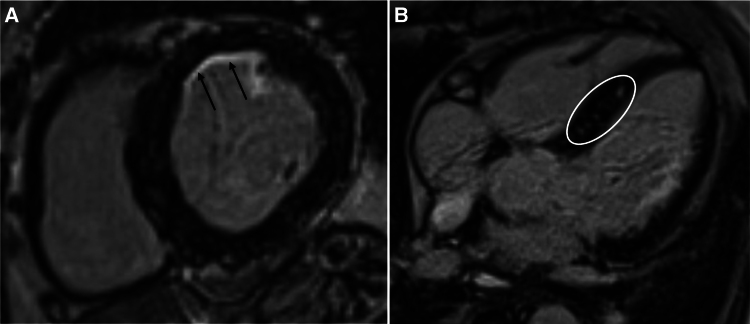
Cardiac magnetic resonance imaging (CMRI). (*A*) Late gadolinium enhancement short-axis view shows subendocardial enhancement of <50% of the anterior wall (arrows). (*B*) The four-chamber view indicates presence of prominent septal arterial collaterals (circle).
